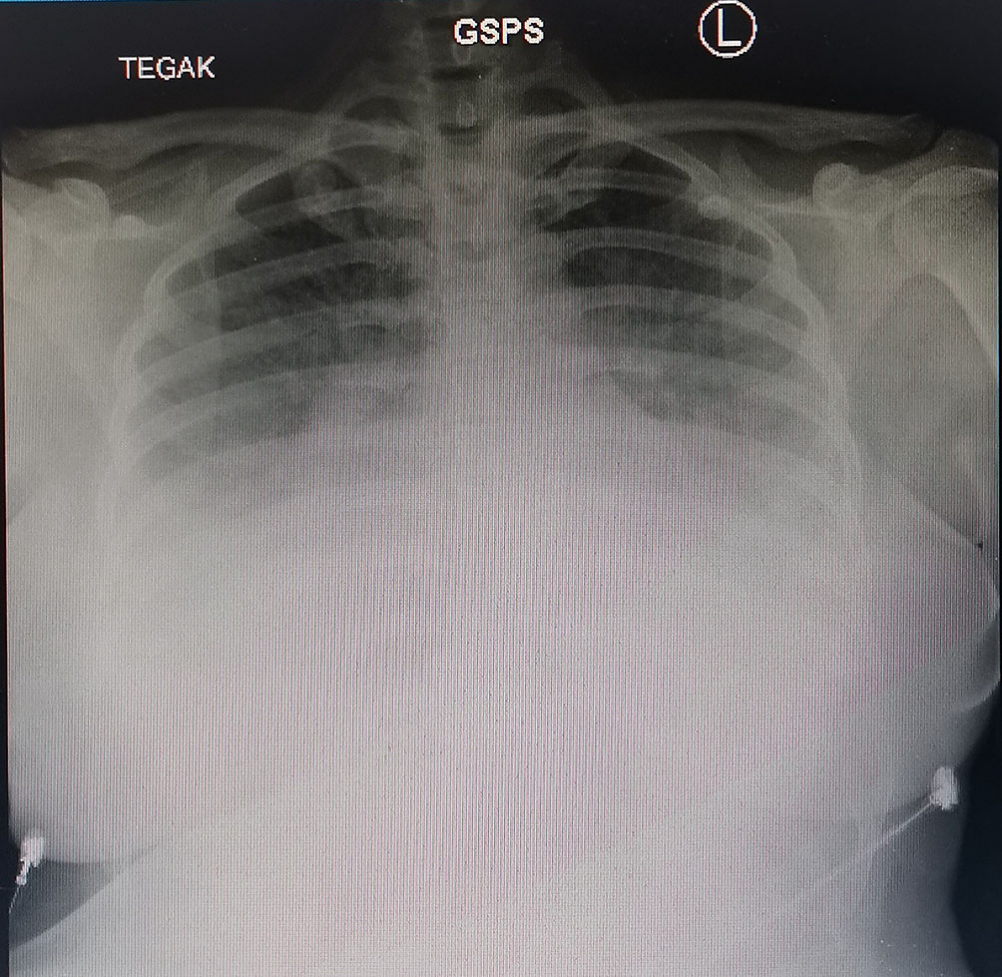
The patient's chest x-ray in the upright position.
